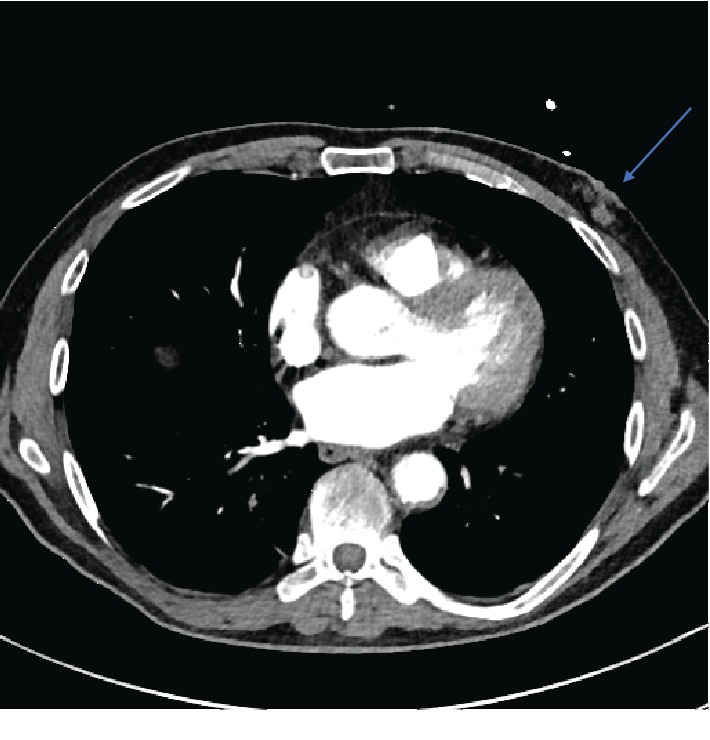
Angio-CT showing a left mammary mass (blue arrow).
